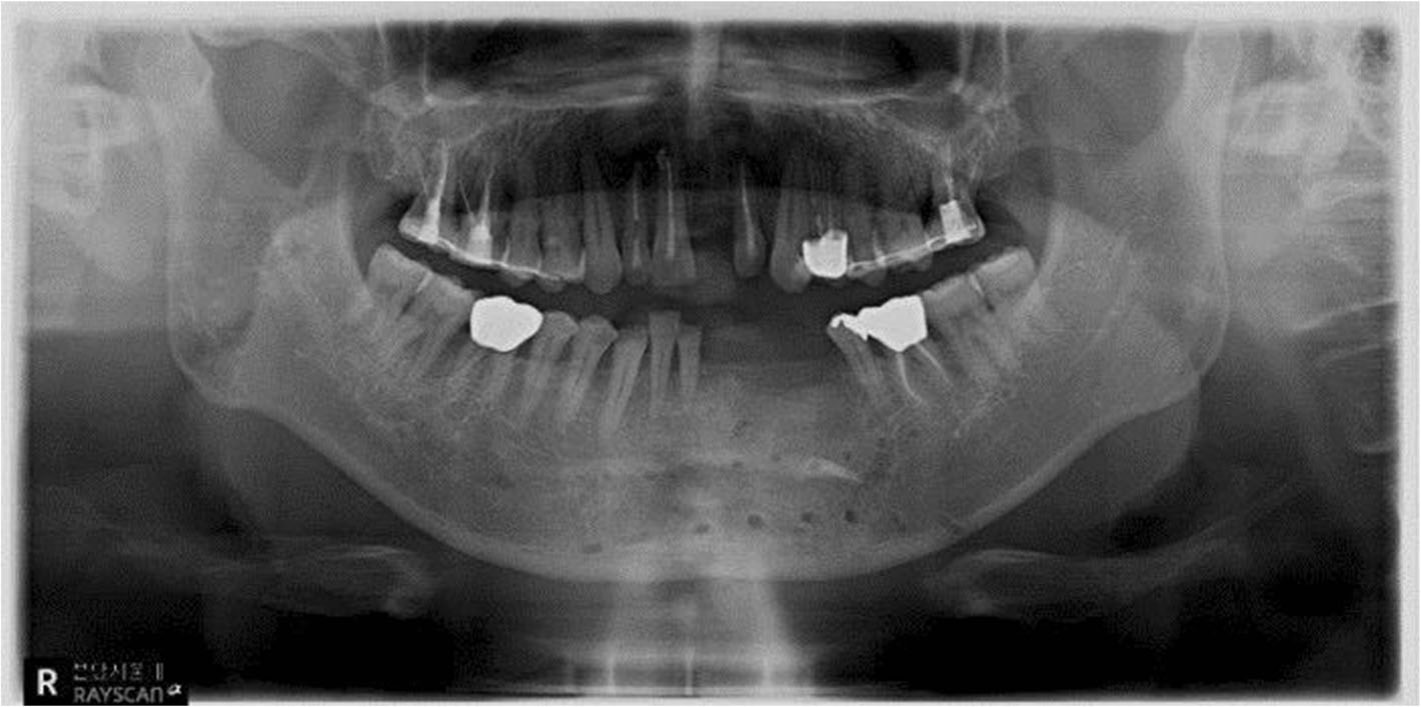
Postoperative panoramic radiograph
